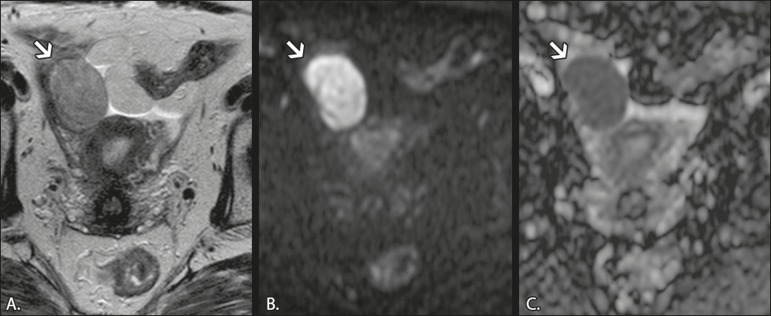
MRI of a 67-year-old female patient with thecoma. A:
